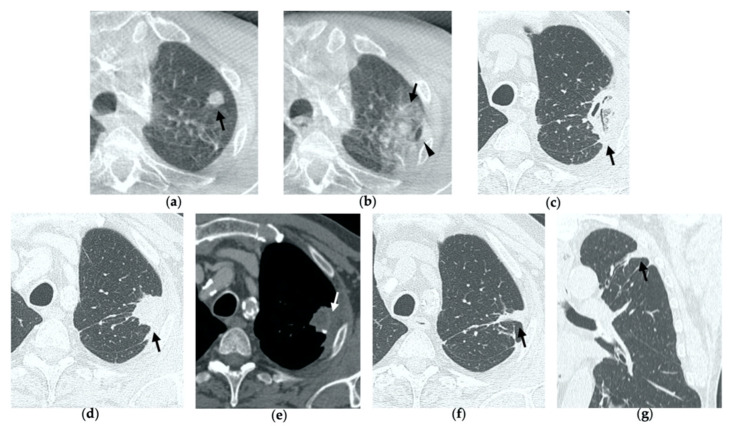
74-year-old man with a pulmonary metastasis from bladder urothelial carcinoma. (a) Cone-beam CT image of the left upper lobe metastasis (black arrow) prior MWA. (b) Cone-beam CT image obtained post-procedure shows hazy GGO of the ablation site surrounding the treated nodule (black arrow) and a small layer of lateral pneumothorax (arrowhead). (c) Axial 1-month follow-up CT image shows a large consolidation with inner cavitation (black arrow). (d,e) Axial 3-month follow-up CT image shows resolution of the cavitation and decrease in size of the consolidation (black arrow) (d) and demonstrates peripheral mild enhancement with no central contrast material uptake (white arrow) (e–g). Axial (f) and coronal (g) CT images obtained after 10 months show a residual fibrotic band (black arrow).
